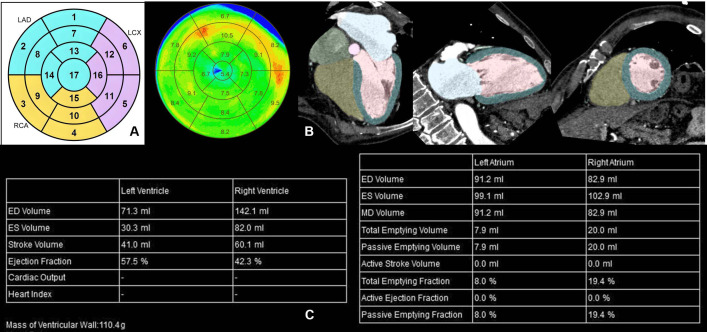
Representative images and derived CCTA parameters. (A) The 17-segment model and LV wall thickness measurements. (B) Four-chamber, short-axis, and two-chamber views showing automatic segmentation of endocardial and epicardial contours. (C) CCTA-derived parameters obtained after correction on the UNITED IMAGING post-processing workstation (LVM, LVMDV, LVMSV, LAMDV, LAMSV, LVEF). LAD, left anterior descending artery; LCX, left circumflex artery; RCA, right coronary artery; ED, end-diastolic; ES, end-systolic; MD, mid-diastolic; CCTA, coronary computed tomography angiography; LVM, left ventricular mass; LVMDV, left ventricular mid-diastolic volume; LVMSV, left ventricular mid-systolic volume; LAMDV, left atrial mid-diastolic volume; LAMSV, left atrial mid-systolic volume; LVEF, left ventricular ejection fraction.
